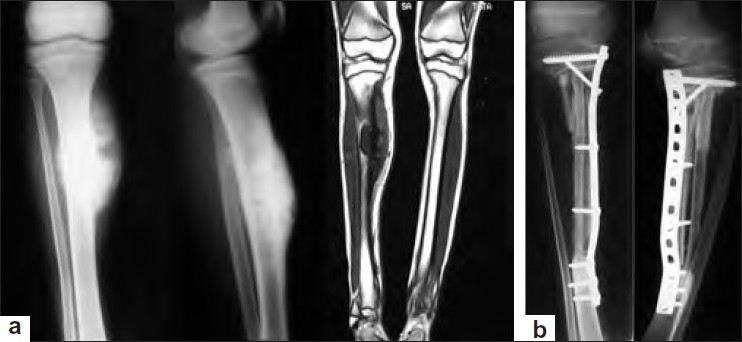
(a) Anteroposterior and lateral radiographs with T1-weighted coronal section of MRI showing Ewing’s sarcoma of proximal one-third diaphysis of right tibia. (b) Anteroposterior and lateral radiographs of right leg showing intercalary reconstruction with a well-incorporated fibular autograft of proximal one-third diaphysis of right tibia at 5-year followup
